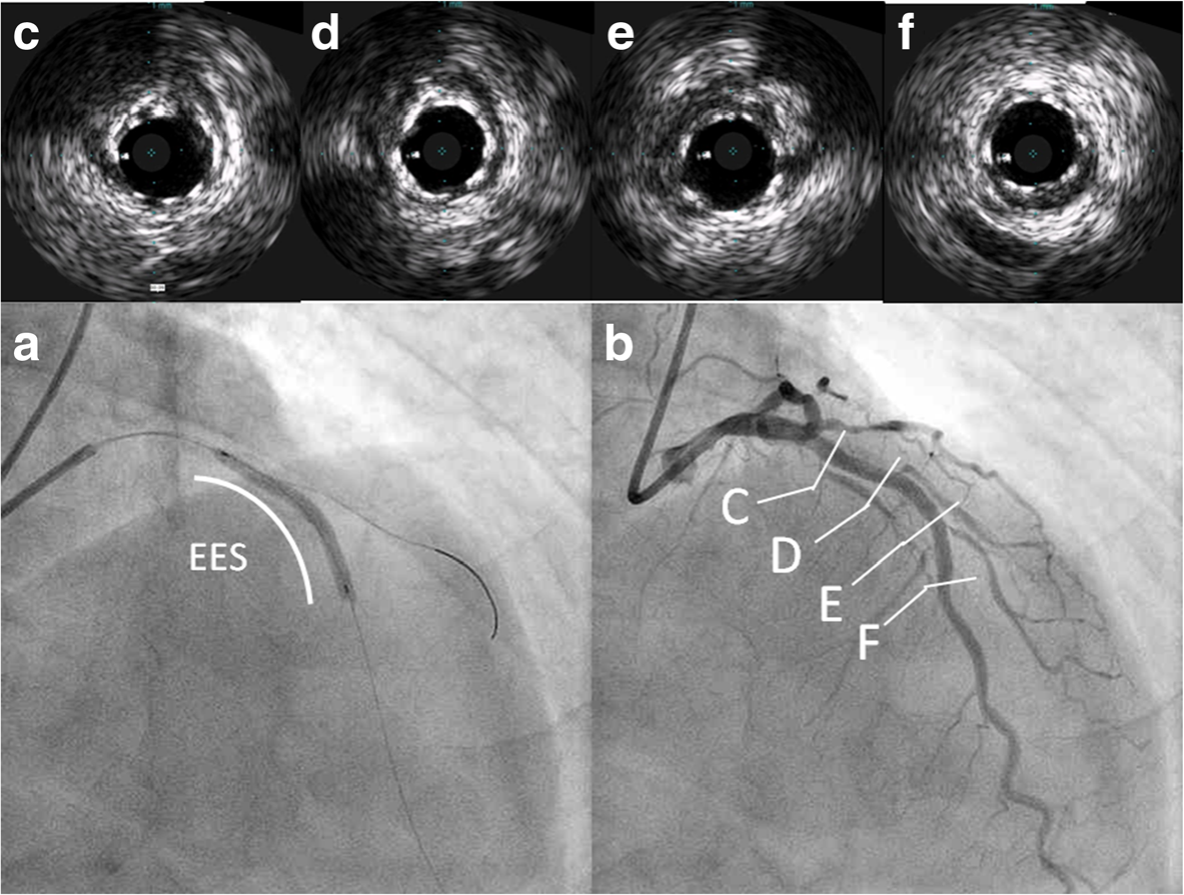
Elective percutaneous coronary intervention to the left ascending artery (a and b) and intravascular ultrasound findings (c-f). a Implantation of an everolimus-eluting stent (3.25 mm in diameter, 33 mm in length). b Final angiogram after post-dilatation, indicating good blood flow. c-f Intravascular ultrasound findings of the implanted everolimus-eluting stent (c proximal part of the stent, d part of first diagonal branch, e part of second diagonal branch, f distal part of the stent) indicating that the stent was well-expanded and without incomplete apposition
